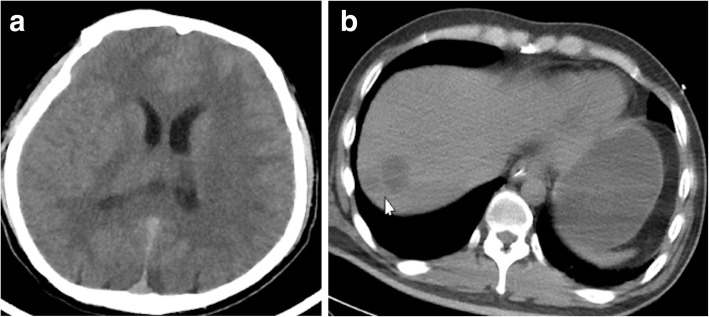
a: Head CT scan showed diffuse cerebral edema; b: Abdominal CT scan demonstrating an area of abnormal attenuation measuring 24 mm × 19 mm in the right lobe of the liver, suggestive of a single abscess
